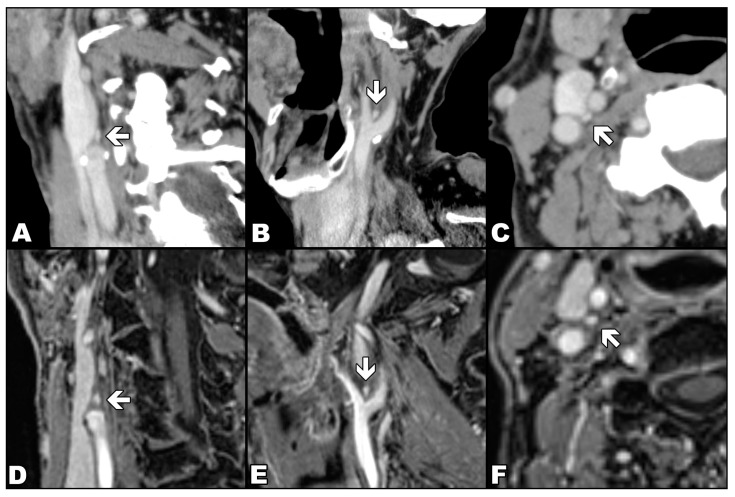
Comparison of the appearance of the carotid body (arrows) in contrast-enhanced computed tomography (upper row) with the appearance in contrasted-enhanced VIBE sequence in T1-weighted image with Dixon technique in the water-only images (lower row). Computed tomography (images (A–C)) and magnetic resonance (images (D–E)) examinations show the carotid body in three orthogonal planes: oblique coronal (A,D), oblique sagittal (B,E) and axial (C,F).
